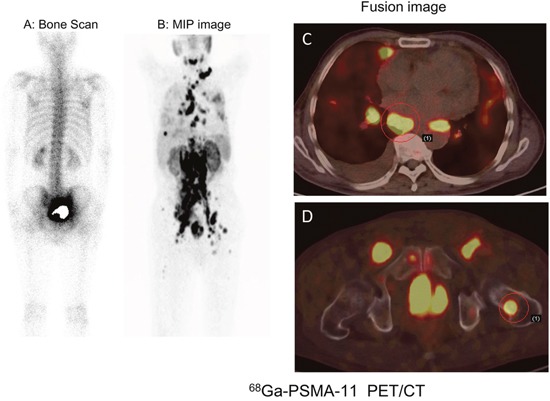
68Ga-PSMA-11 PET/CT versus bone scan for the evaluation of multiple metastases in a 73-year-old patient with right leg edema Besides the primary prostatic lesion, multiple PSMA-avid lesions in lymph node and bone metastases were detected on ^68^Ga-PSMA-11 images. A. Bone scan. B, C, D. ^68^Ga-PSMA-11 images: B, MIP image; C, thoracic fusion image; D, Pelvic fusion image.
